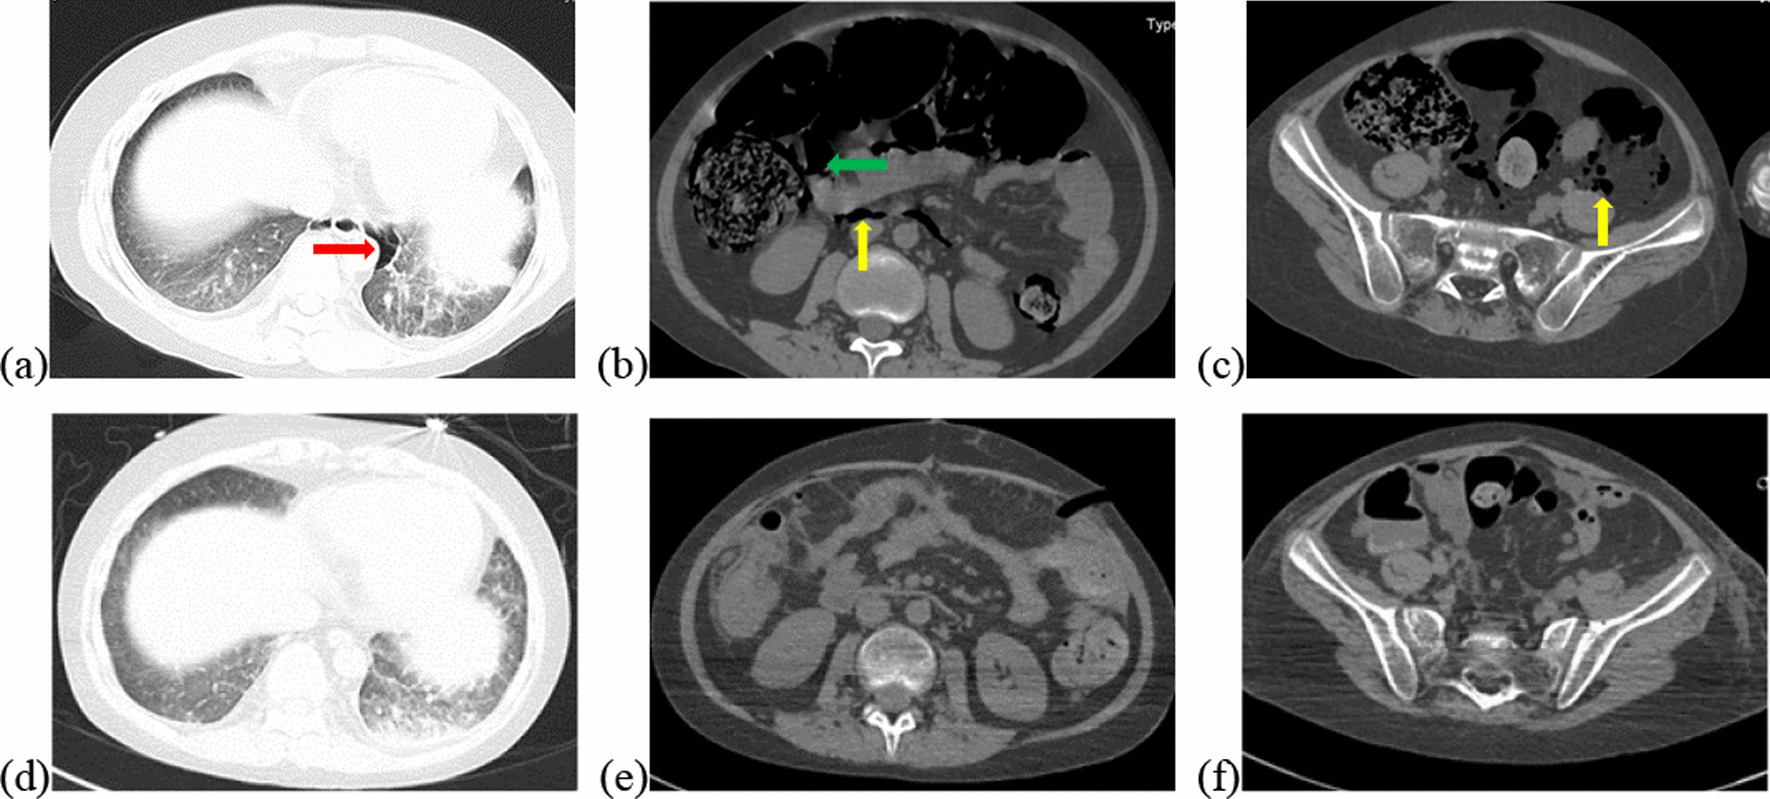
Initial abdominal CT and one week later. Initial abdominal CT scan showed massive free gas collected in the colon wall denoted by the green arrow (b). The gas could even be observed in the mediastinum in the lung view (a) denoted by the red arrow, retroperitoneal space in the abdomen (b) and pelvic cavities view (c) denoted by the yellow arrows. Free accumulated gas was gradually absorbed after one week of conservative treatments in the same view (d–f)
